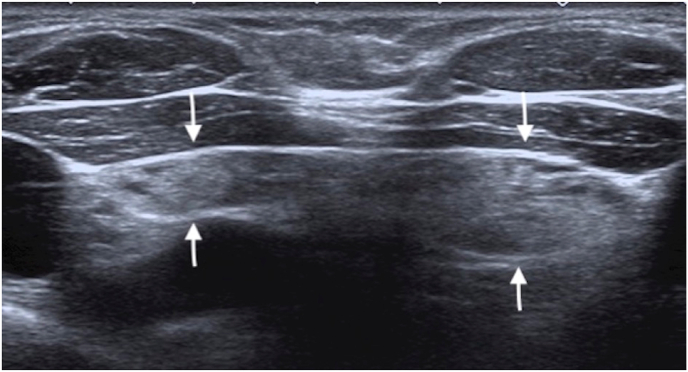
The ultrasonography image shows the fatty replacement of the thymus (arrows) in a 10-year-old girl.
